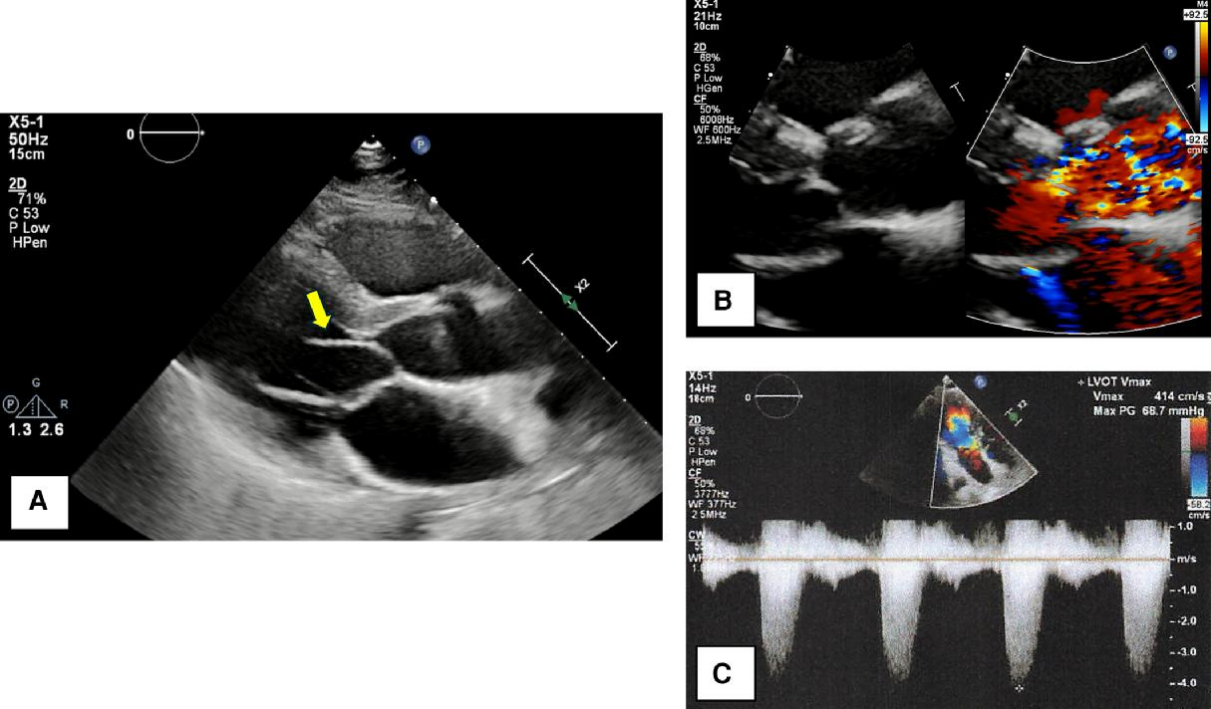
Transthoracic echocardiogram findings. (*A*) The high-echoic linear structure abutting the interventricular septum during systole was observed in long axis view of left ventricle (yellow arrow). (*B*) Zoomed image around the aortic valve in the left ventricular long-axis view. The accelerated blood flow in the LVOT with mosaic coloured Doppler was observed. (*C*) Pulse-wave Doppler image showing blood flow in the left ventricular outflow tract. The maximum flow velocity was 4.1 m/s.
